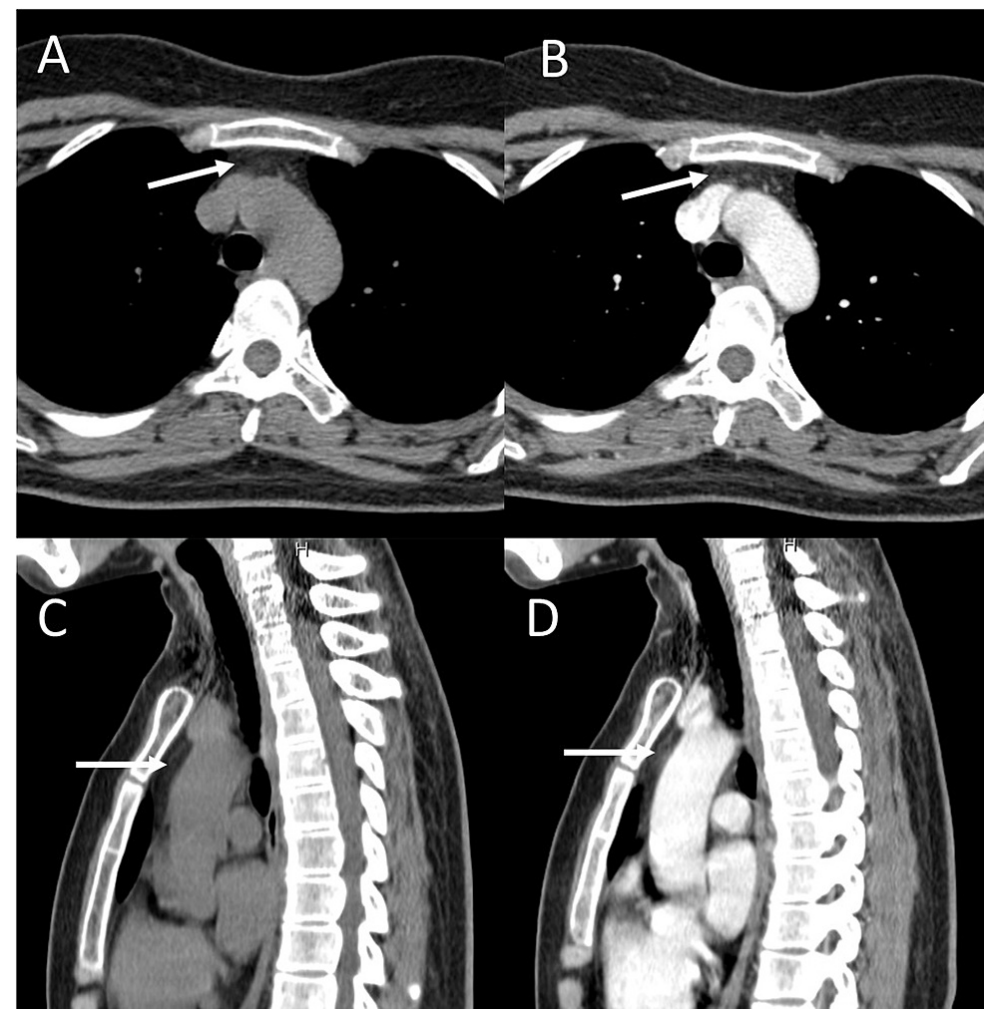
MDCT of the chest (A) Axial non-contrast, (B) axial with contrast, (C) sagittal non-contrast, and (D) sagittal with contrast views show prominent soft tissue without abnormal enhancement at mid-superior mediastinum in the prevascular space anterior to the great vessels (arrow). MDCT, Multidetector computed tomography.
